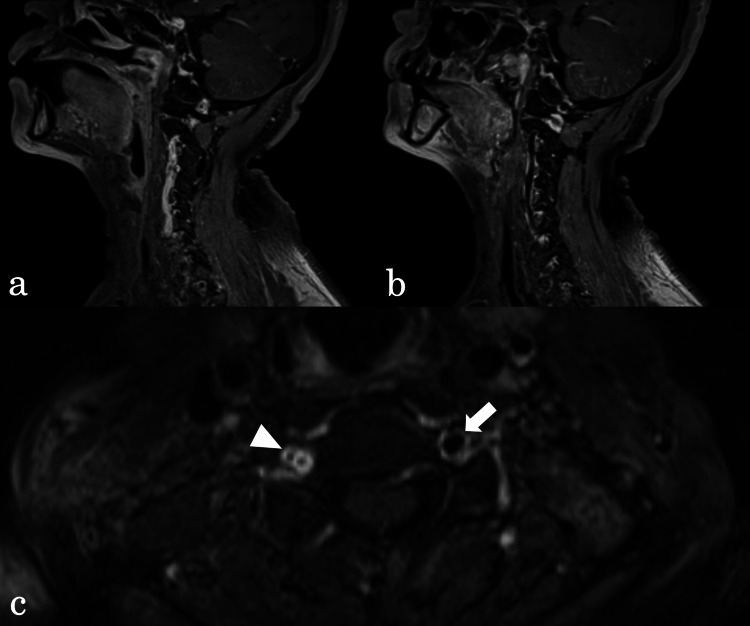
Contrast-enhanced T1-weighted MRI (a) The right vertebral artery (VA) showing dissection. (b) The left VA showing normal findings. (c) In contrast to the normal left VA (white arrow), the right VA (white arrowhead) showed double-barrel findings due to dissection.
